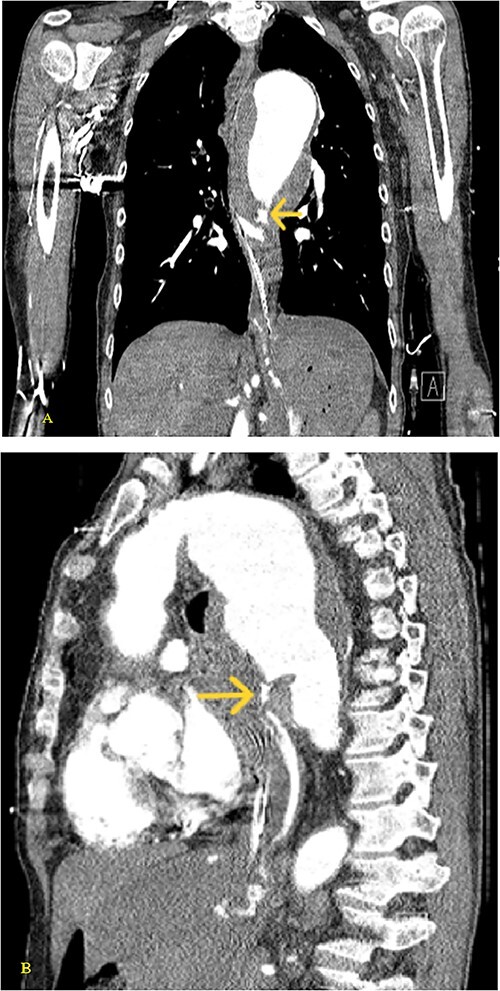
(A) Coronal and (B) sagittal views of the arterial phase CT chest with IV contrast performed during the initial trauma evaluation show aneurysmal dilation of the thoracic aorta with contrast extravasation into the esophagus and stomach.
